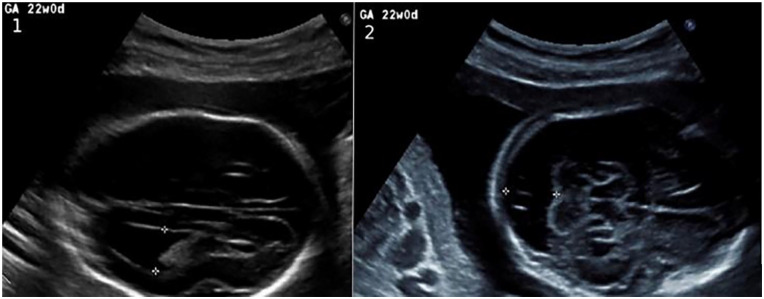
Fetus 2: ultrasound images. Fetus 2 with (1) dilated lateral ventricles of 12 mm and (2) mega cisterna magna of 18.5 mm.
